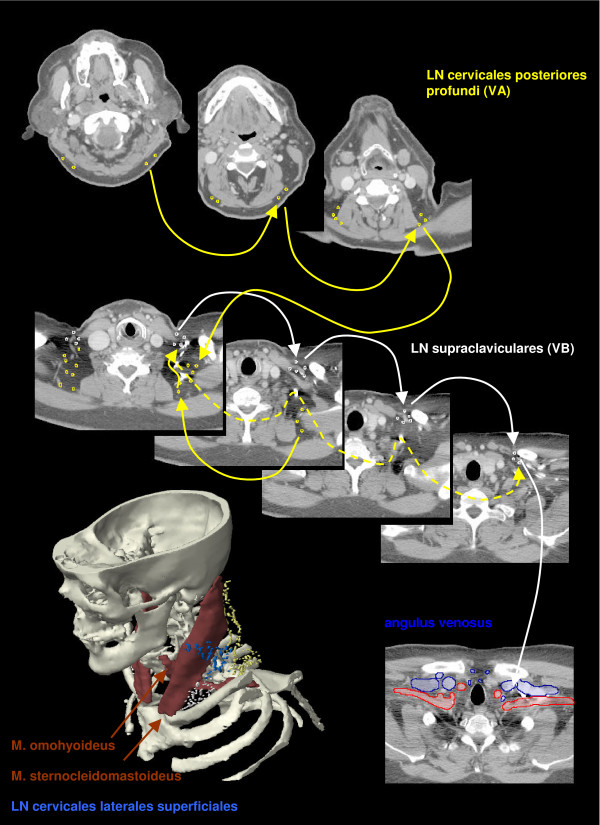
Lymph regions and drain contoured in transversal CT slices: LN cervicales posteriores profundi (yellow) and LN supraclaviculares (white).
